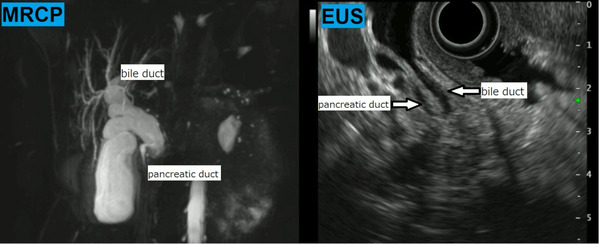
Magnetic resonance cholangiopancreatography and endoscopic ultrasound confirmed bile duct dilation without findings of pancreaticobiliary maljunction
